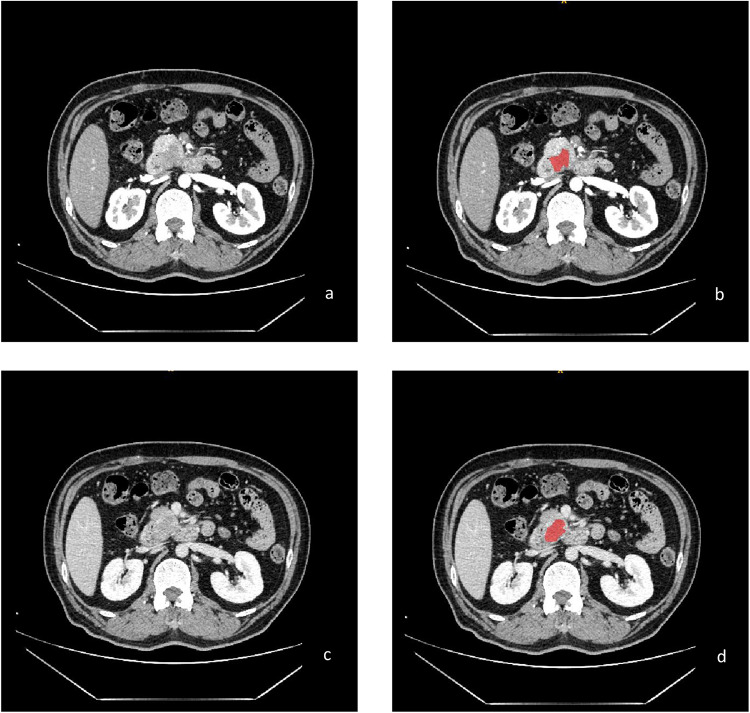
Enhanced CT images of moderately differentiated PC in the arterial phase (a) and venous phase (c); the treatment ROI using ITK-SNAP software (b,d).
